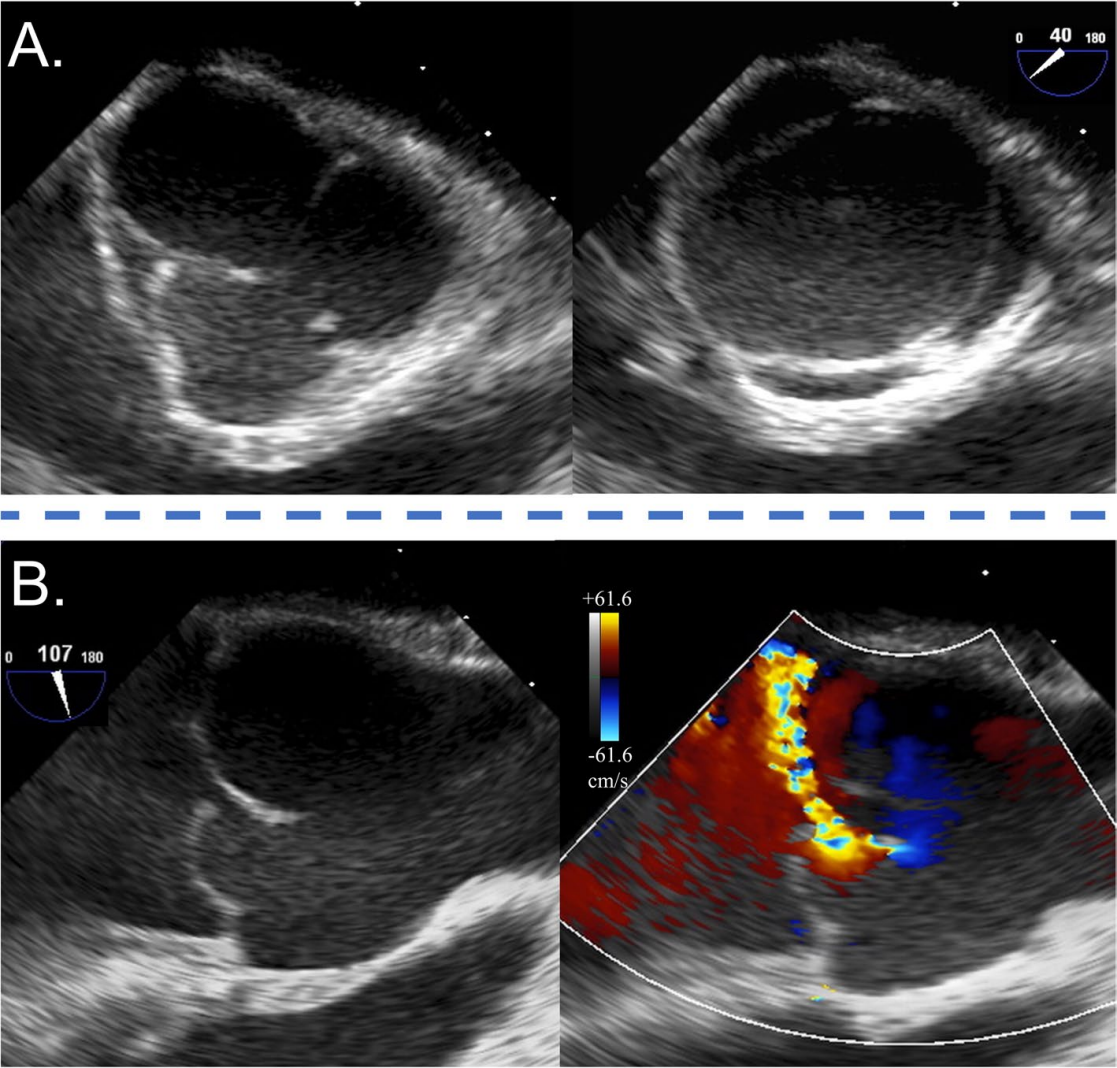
Preoperative transesophageal echocardiography. A Short-axis imaging in diastolic and systolic phases. B Long-axis B-mode and color Doppler imaging during the diastolic phase
